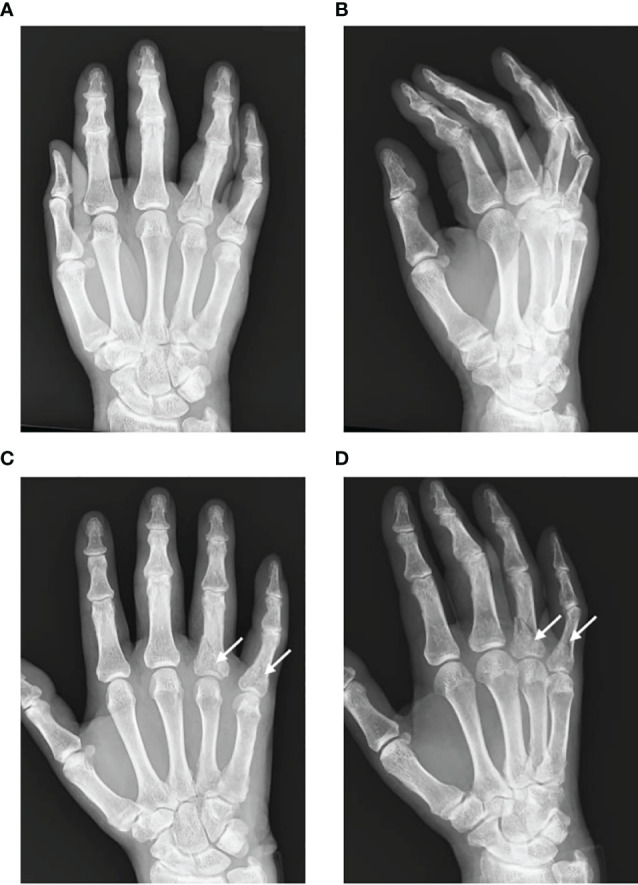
Referential X-ray images in a patient with CRPS following phalanx fractures. The patient underwent surgical treatment for fractures of the right fourth and fifth proximal phalanges and was diagnosed with CRPS due to persistent throbbing pain, swelling of the fingers, heat sensation, abnormal sweating in the palm, and a limited range of motion of the fourth and fifth fingers. (A, B) Radiographic images at the time of the injury. (C, D) Radiographic images at 5 months postoperatively. White arrows indicate localized patchy bone atrophy, which is not commensurate with the ordinary course of fracture healing.
